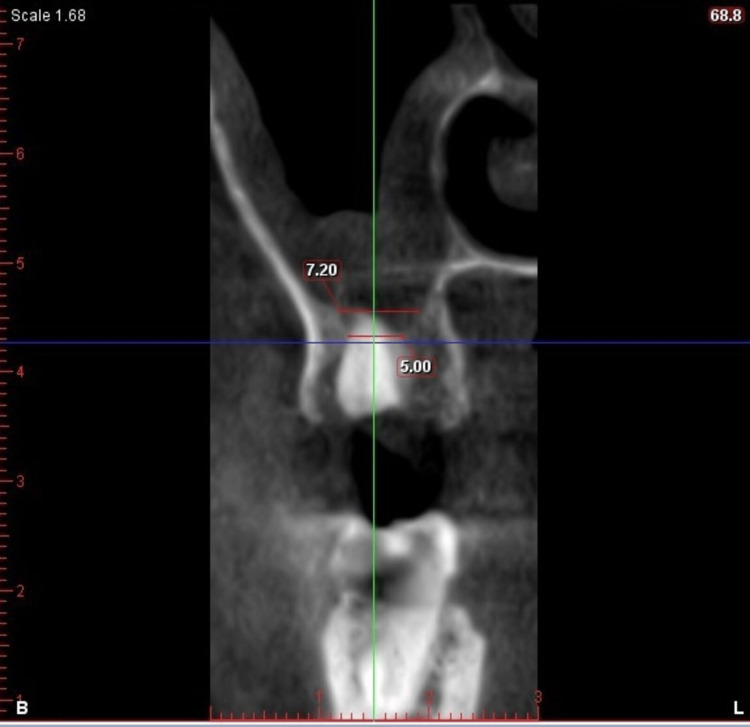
Preoperative cone-beam computed tomography image: paraxial slice (Case 2)
